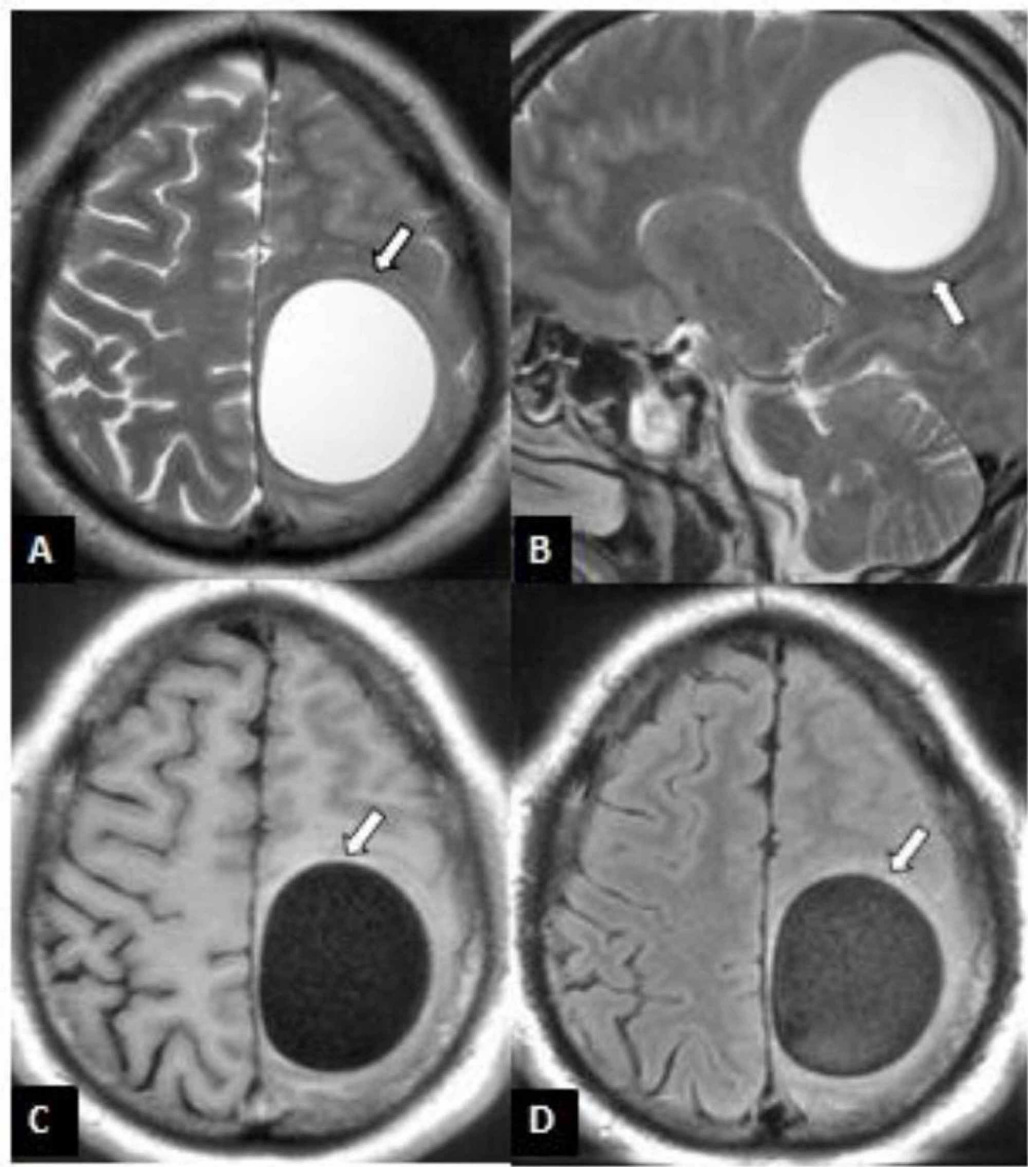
MRI brain of a 28-year-old patient with cerebral hydatid cyst A: Axial T2WI; B: Sagittal T2WI; C:Axial T1WI; D: Axial FLAIR image demonstrating a large uniloculated hydatid cyst (white arrows) in the left fronto-parietal region with minimal overall mass effect relative to size of cyst MRI: magnetic resonance imaging; T2WI: T2 weighted image; T1WI: T1 weighted image; FLAIR: fluid-attenuated inversion recovery
